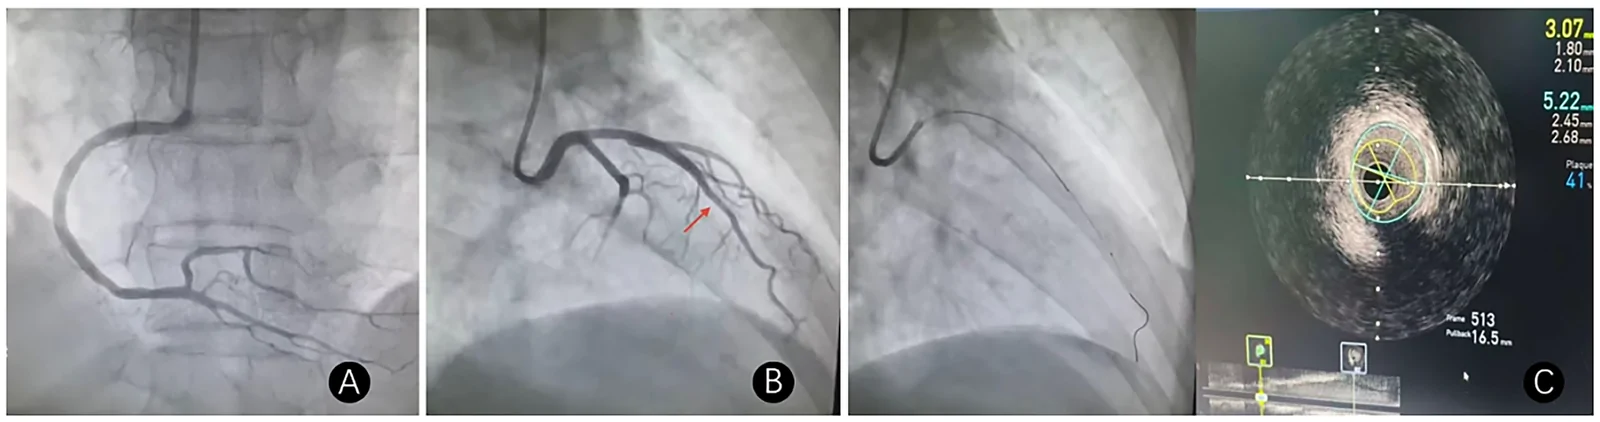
Coronary angiography and intravascular ultrasound (IVUS) findings. (A) No significant stenosis observed in the right coronary artery. (B) Coronary angiography showing 50% stenosis in the mid-segment of the left anterior descending (LAD) artery, with a suspected thrombus shadow. (C) IVUS examination revealing a minimum lumen area of 3.07 mm2 and a plaque burden of 41% in the mid-LAD segment.
